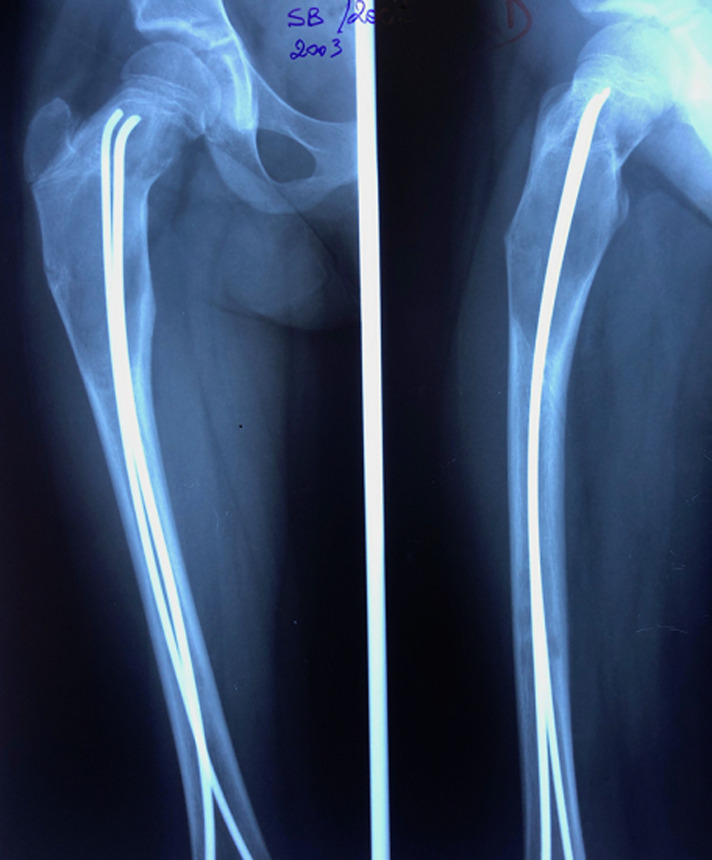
image radiographique d´un enclouage par deux broches de Metaizeau sur une lésion de dysplasie fibreuse
